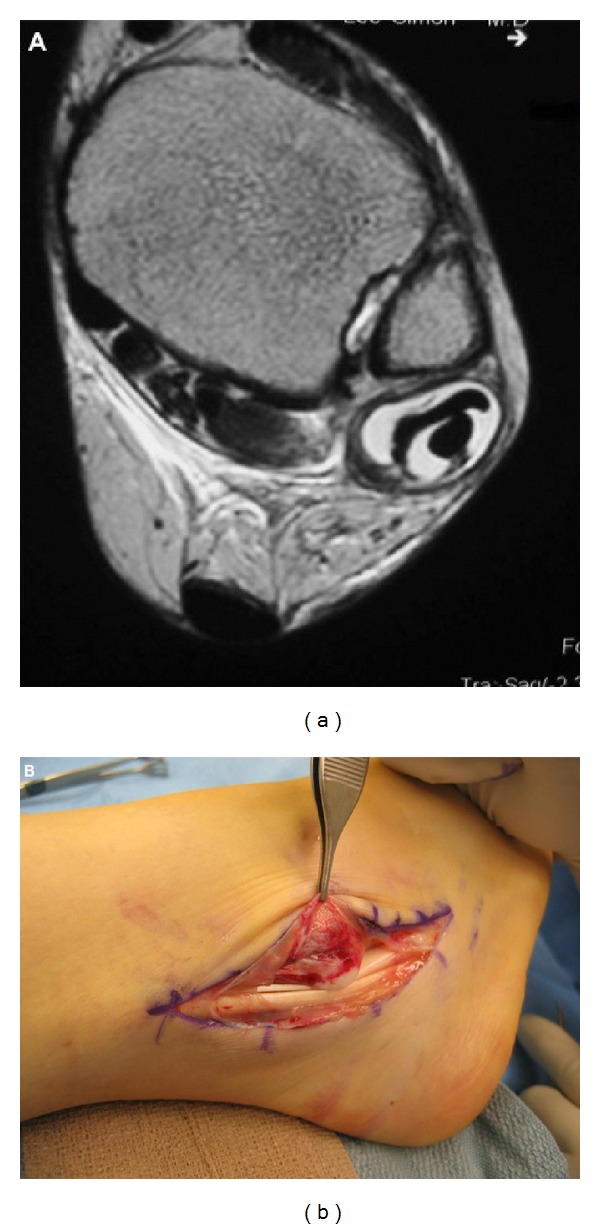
(a) T2-weight axial MRI image demonstrating peroneal tendon tendonitis (note the hyperintense (white) edema surrounding the hypointense (black) peroneal tendons); (b) intraoperative photograph demonstrating a lateral approach to the ankle and exposure of the peroneal longus and brevis tendons.
